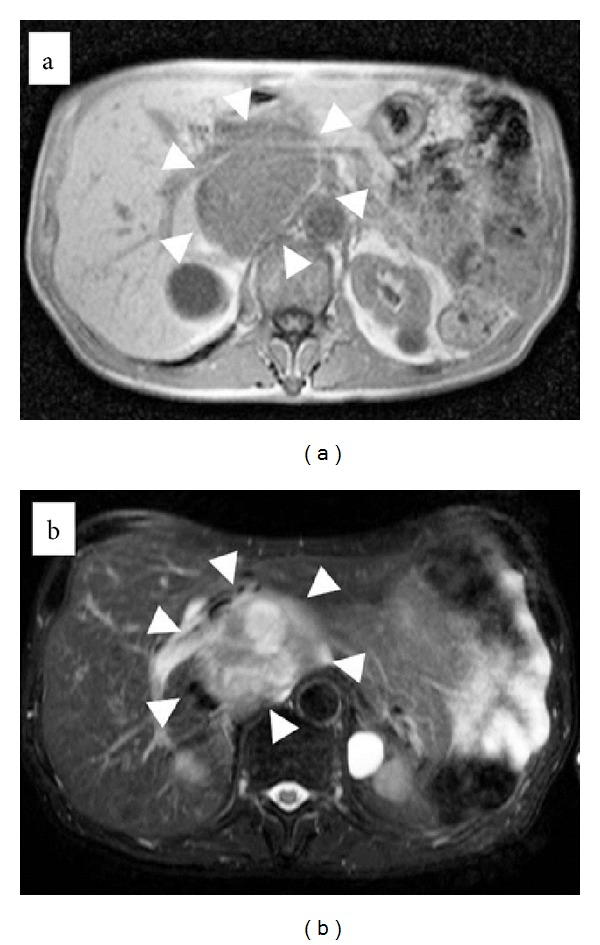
Magnetic resonance imaging (MRI) of the abdomen. Magnetic resonance imaging (MRI) of this tumor revealed a contrasting low intensity on the T1-weighted image ((a) arrow head) and high intensity on the T2-weighted image ((b) arrow head).
